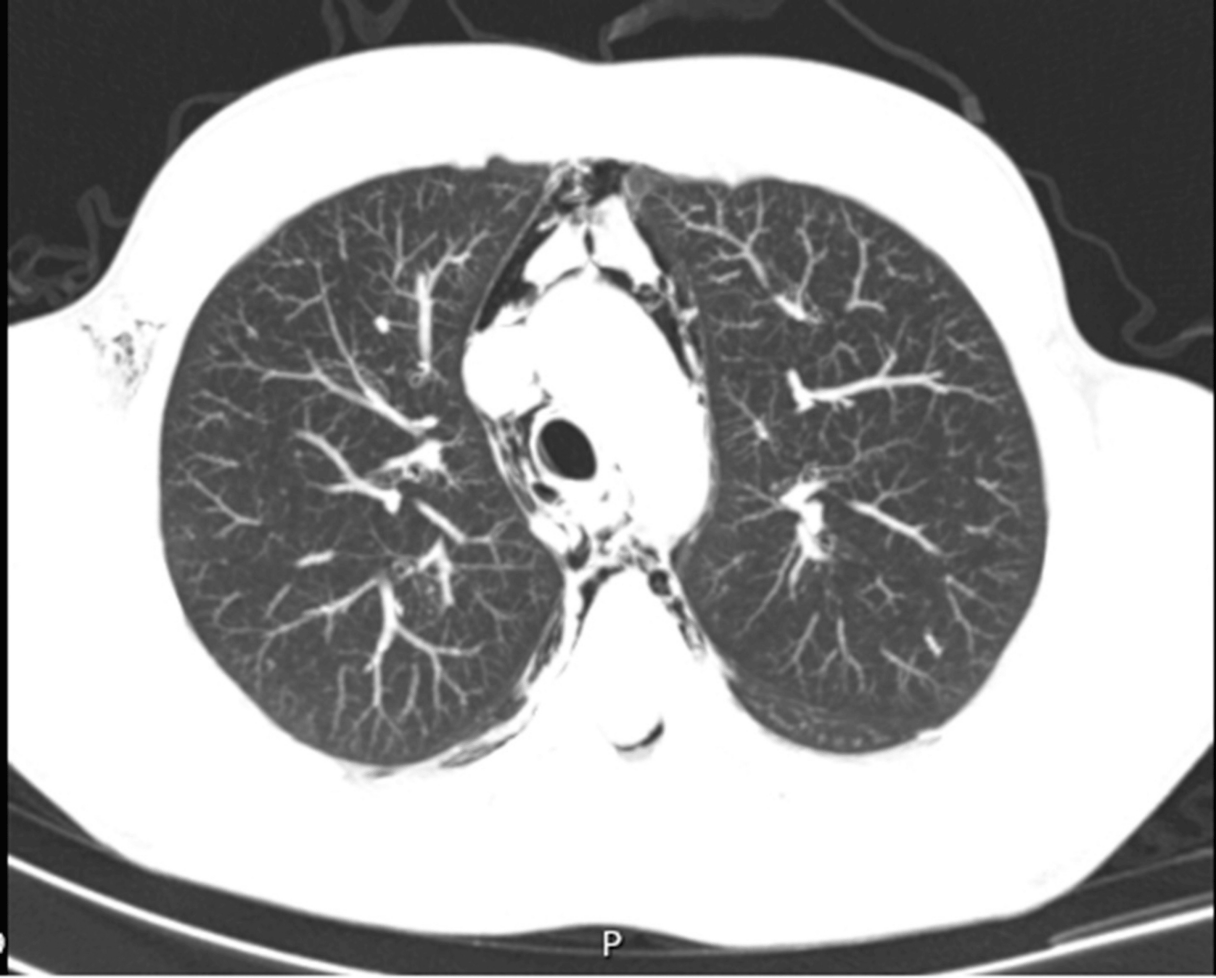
CT scan revealing pneumomediastinum.
